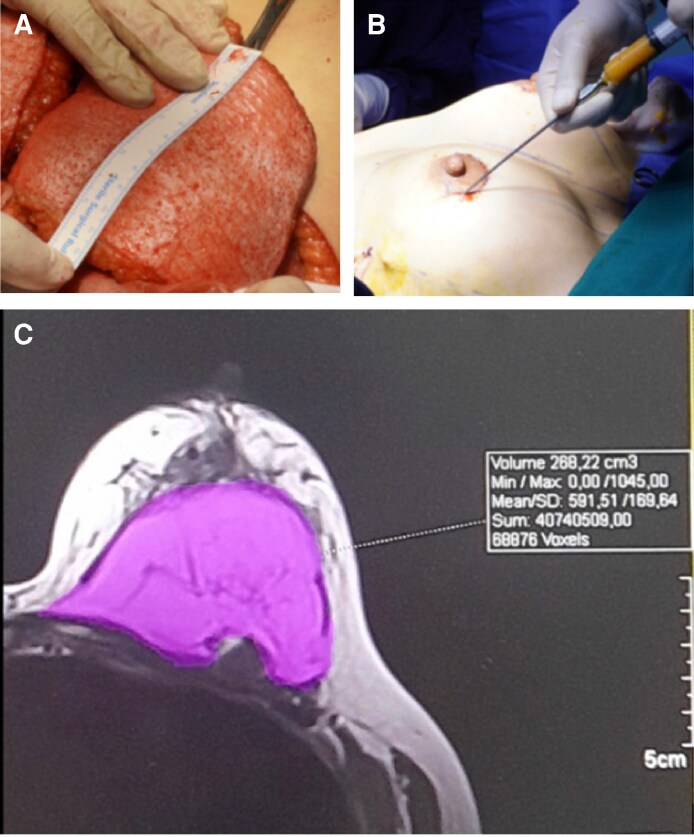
Volumetric assessment in autologous breast augmentation. Intraoperative evaluation of total breast volume using pedicled TRAM flap measurement—(A) VTRAM and (B) fat grafting with a multi-port cannula. (C) Postoperative MRI volumetry at 6 months demonstrating segmented retained volume of 268.22 cm^3^. TRAM, transverse rectus abdominis myocutaneous; VTRAM, volume of the transverse rectus abdominis myocutaneous flap.
